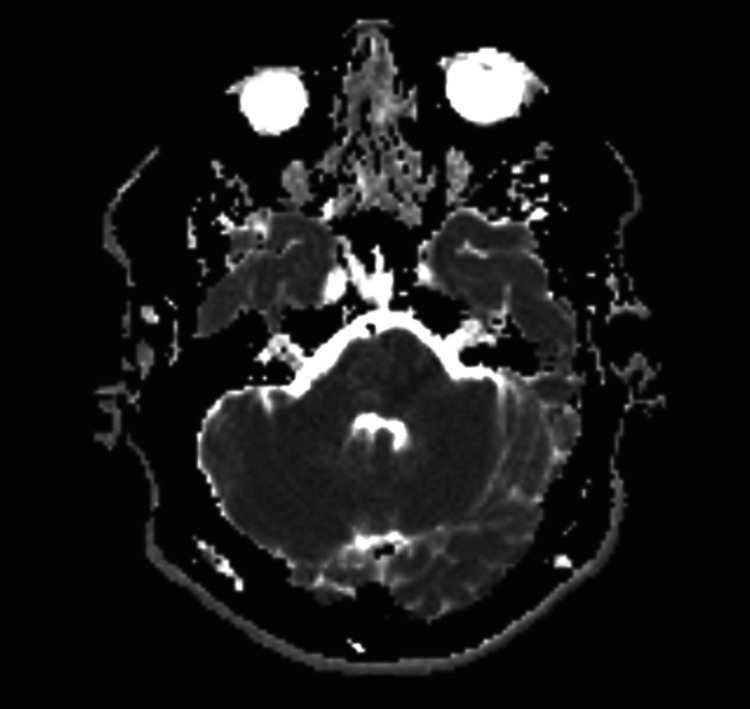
MRI brain ADC view showing left pontine low signal intensity diffusion restriction.
